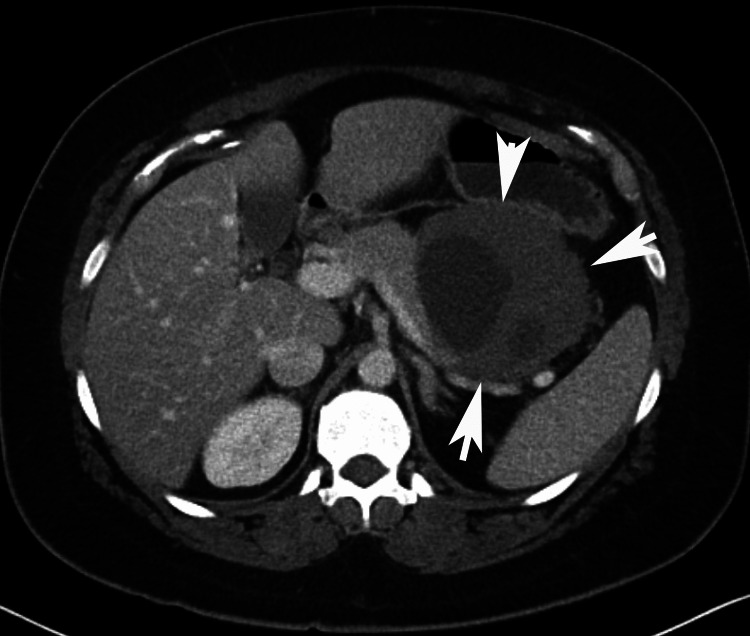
CT image at presentation showing the large tumor The tumor is indicated by white arrowheads.
